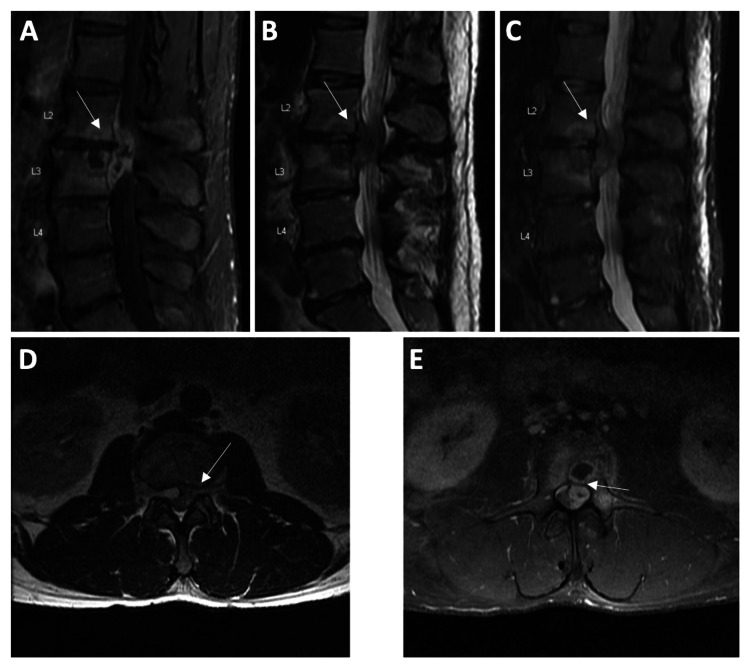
MRI with and without contrast of the lumbar spine. Sagittal T1 with contrast (A), sagittal T2 (B), sagittal T2 STIR (C), axial T1 with contrast (D), and axial T2 (E) at the level of interest. MRI shows enhancing collection at L2-3 ventral and to the left of the thecal sac, causing circumferential compression. The collection measures 38 mm (cranial-caudal) by 14 mm (anterior-posterior) by 13 mm (lateral). The inferior L2 vertebral body, L2-3 intervertebral disc, and superior L3 vertebral body demonstrate heterogenous contrast enhancement. STIR: short TI inversion recovery
